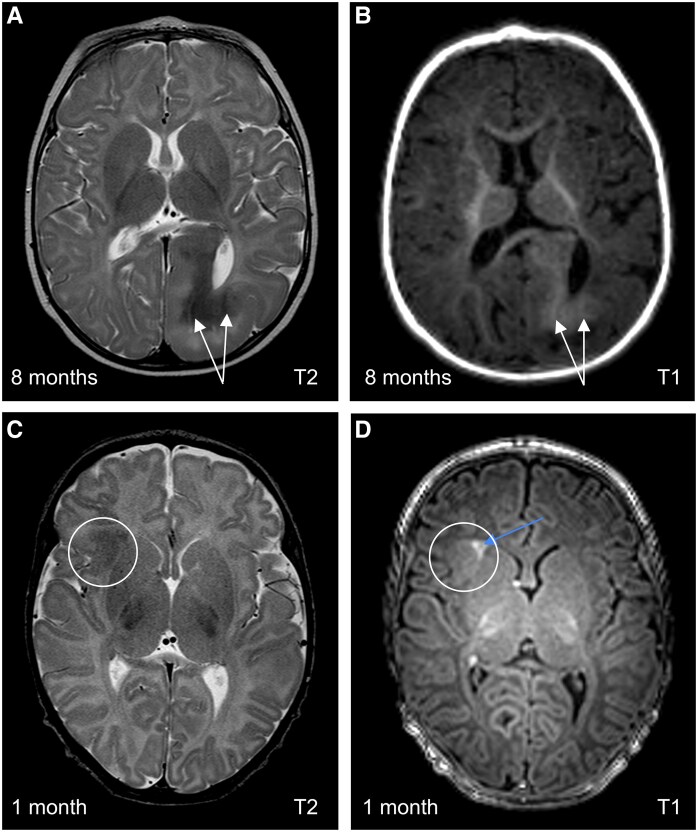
Calcified tuber on unmyelinated MRI. Comparison of the calcified and uncalcified T2-hypointense lesion on axial scans. Patient 23. Calcified lesion in the left occipital lobe. On T2-weighted image (A) calcified areas are noticeably hypointense irregularities compared to surrounding T2-hypointense lesion, hyperintense on T1-weighted image, respectively (B) (white arrows). Patient 27. Lesion in the right frontal lobe is without calcification (circle). T2-weighted image (C) displays homogeneously hypointense lesion, slightly hyperintense on T1-weighted image (D). Strikingly T1 hyperintense transmantle sign (blue arrow) can be seen as well.
